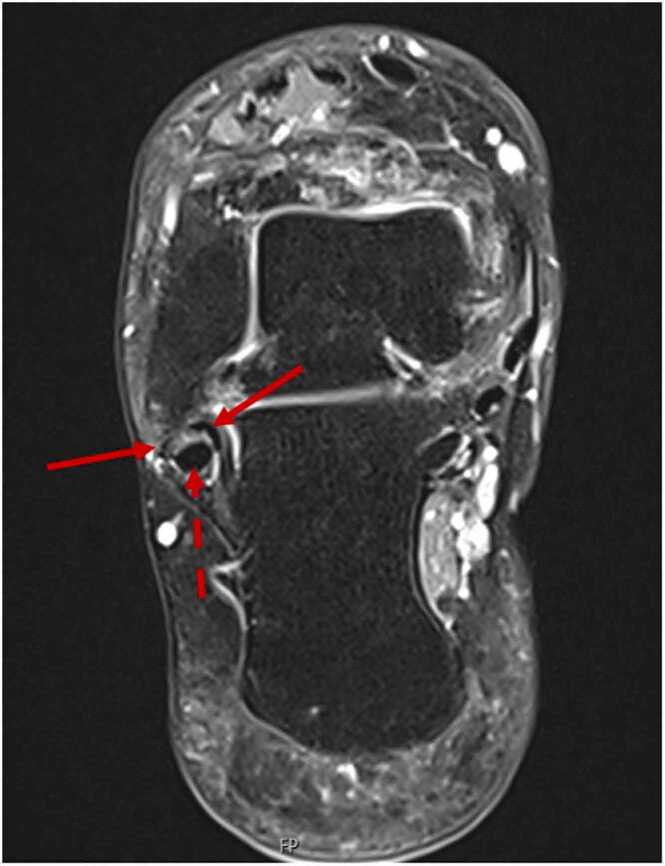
A 44-year-old patient with pain behind the lateral malleolus pain persisting for more than 8 months. The MRI scan, transverse proton density with fat suppression, shows a complete split rupture of the peroneus brevis (solid red arrow). The peroneus longus tendon is normal and is marked with a dashed arrow.
